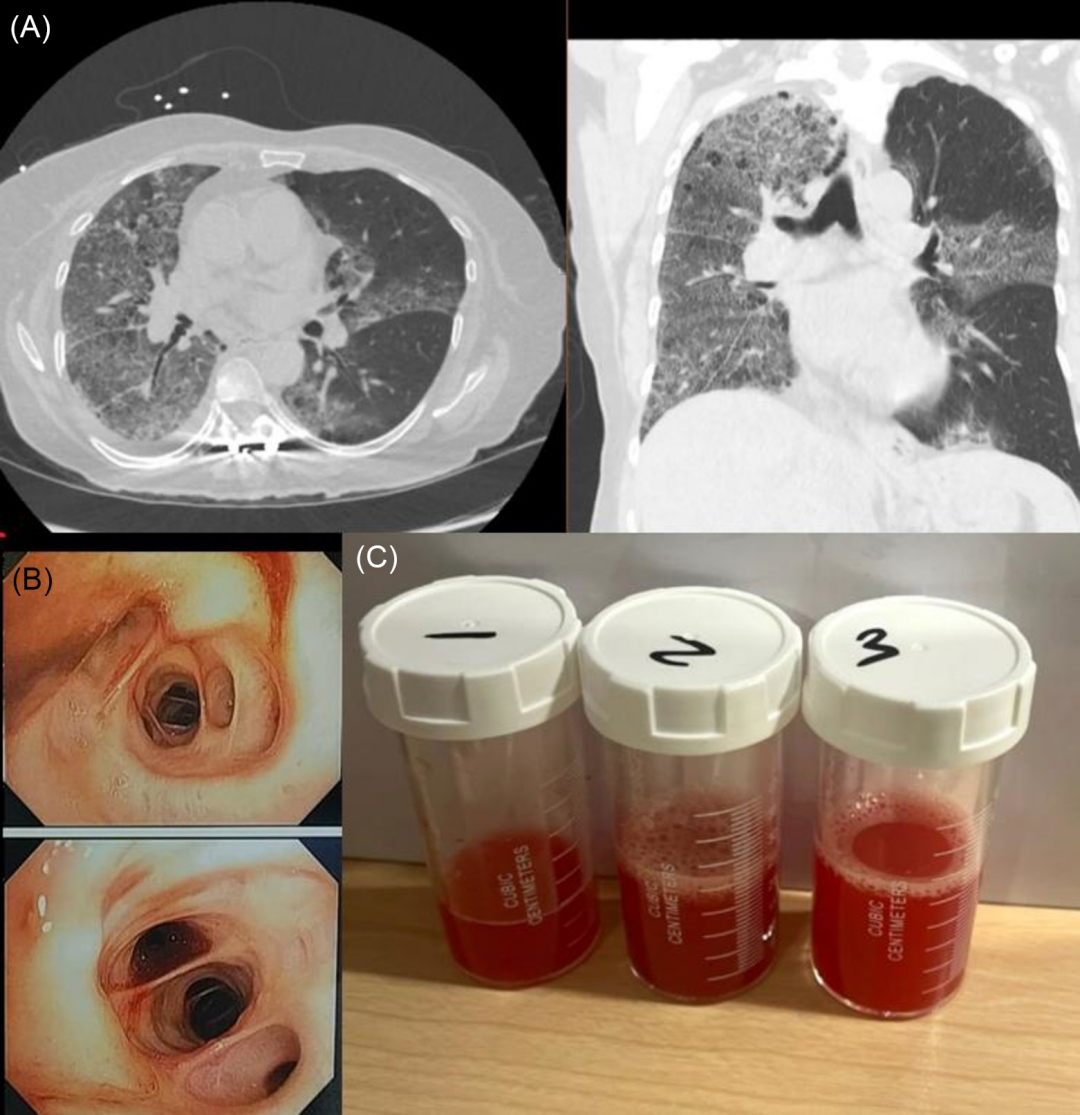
(A) Axial and coronal CT demonstrating diffuse bilateral ground‐glass opacities. (B) Bronchoscopy showing hemorrhagic mucosa. (C) BAL aliquots with progressively bloody appearance consistent with DAH.
